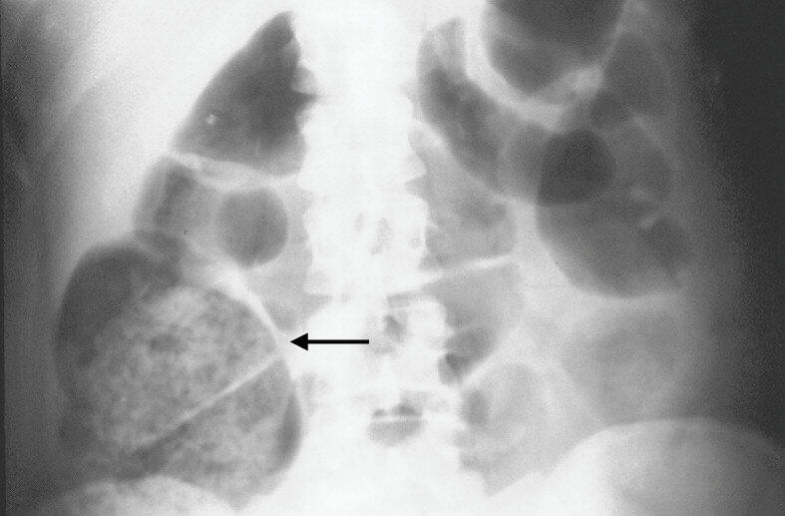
Plain abdominal radiographs of a patient with acute appendicitis. Observe the image of fecal loading in the cecum, which is distended (arrow).
